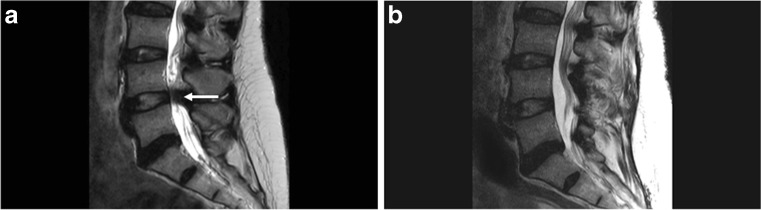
Spinal complications of XLH. a MRI lumbar spine showing significant canal stenosis at L4–5 secondary to ossification of the ligamentum flavum. b Post-operative MRI lumbar spine of the same patient showing significant improvement in the dimensions of the canal after lumbar laminectomy
